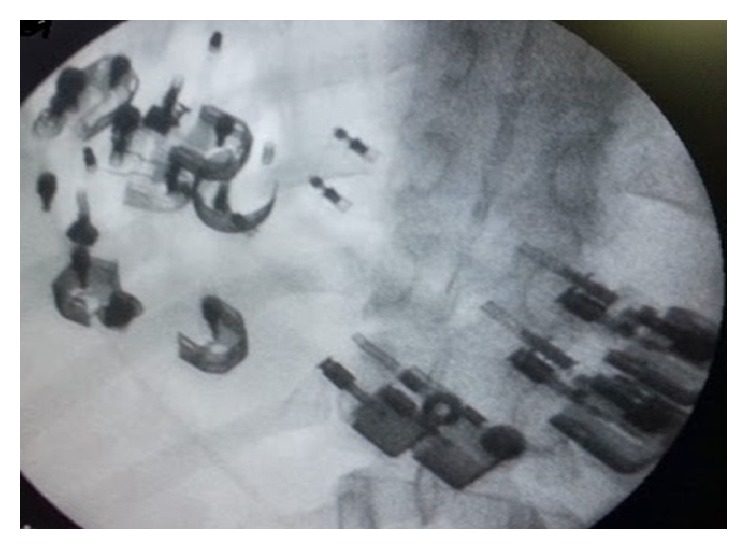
The plain abdominal radiograph showing lighters.
